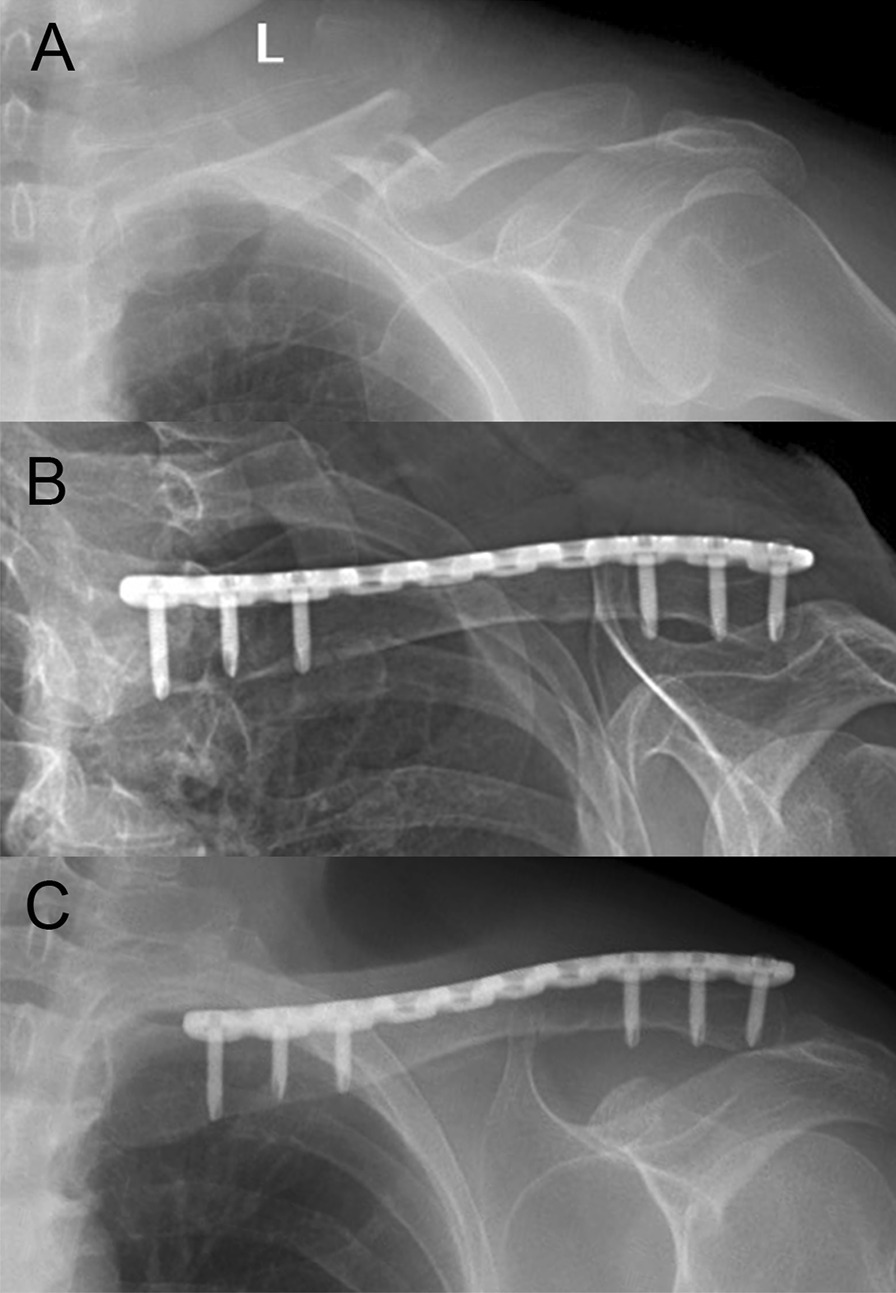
X-ray film of a 55-year-old female involved in a motorcycle accident. A Preoperative X-ray film indicated midshaft clavicle. B Immediate post-op X-ray film showed that the fracture was reduced. C X-ray film at three months after surgery shows that bone union was obtained
